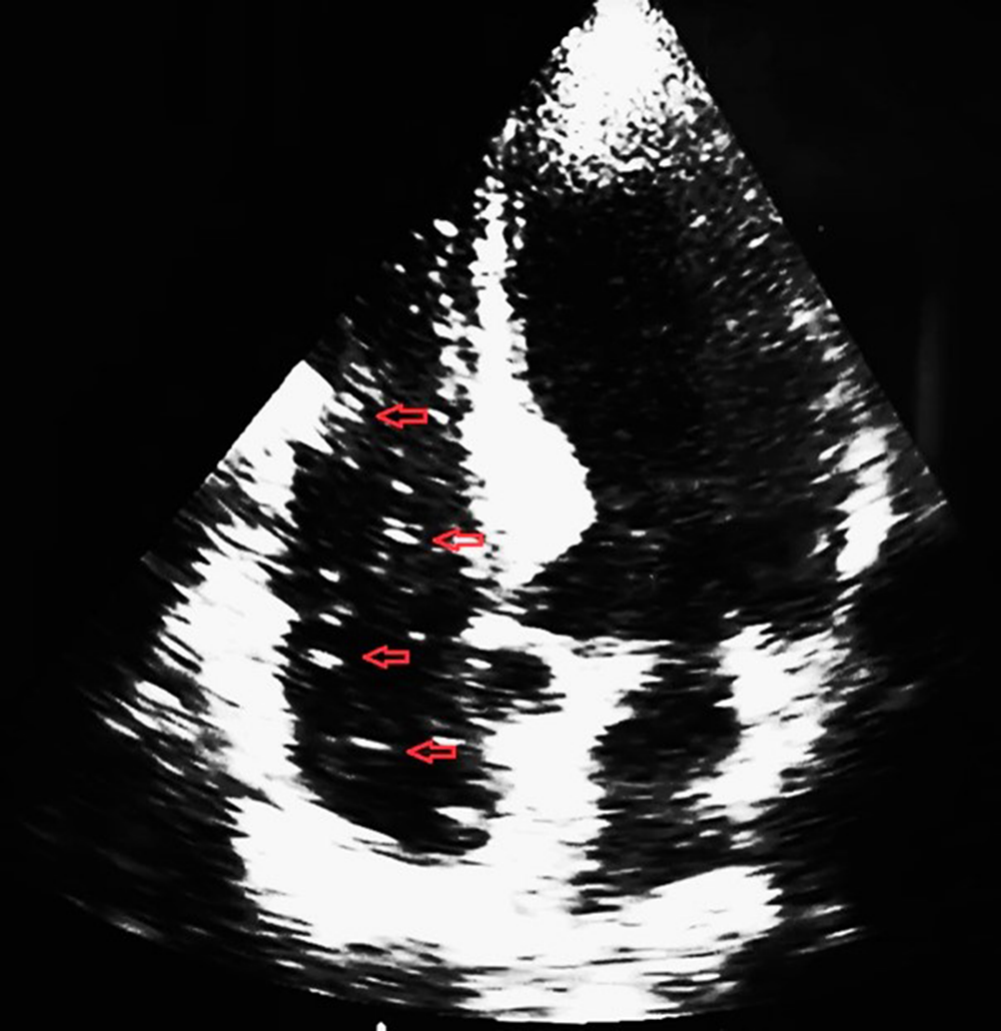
Air bubble in the right ventricle on cardiac ultrasound.
